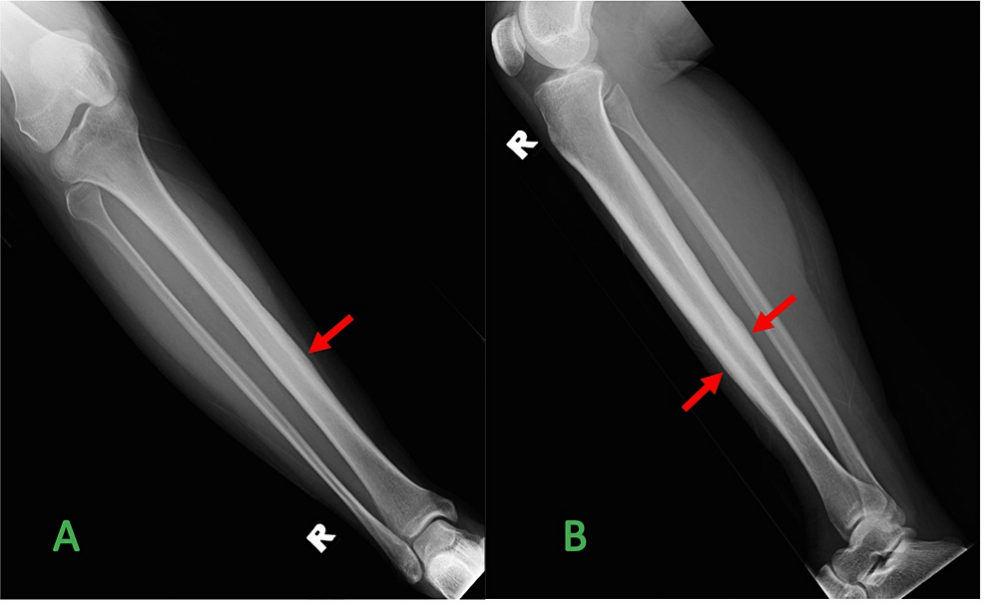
Radiograph of right tibia and fibula A: Frontal projection, B: Lateral projection shows cortical thickening of the shaft of the right tibia (arrows) with a normal cortical outline of the right fibula and the rest of the visualized bones
